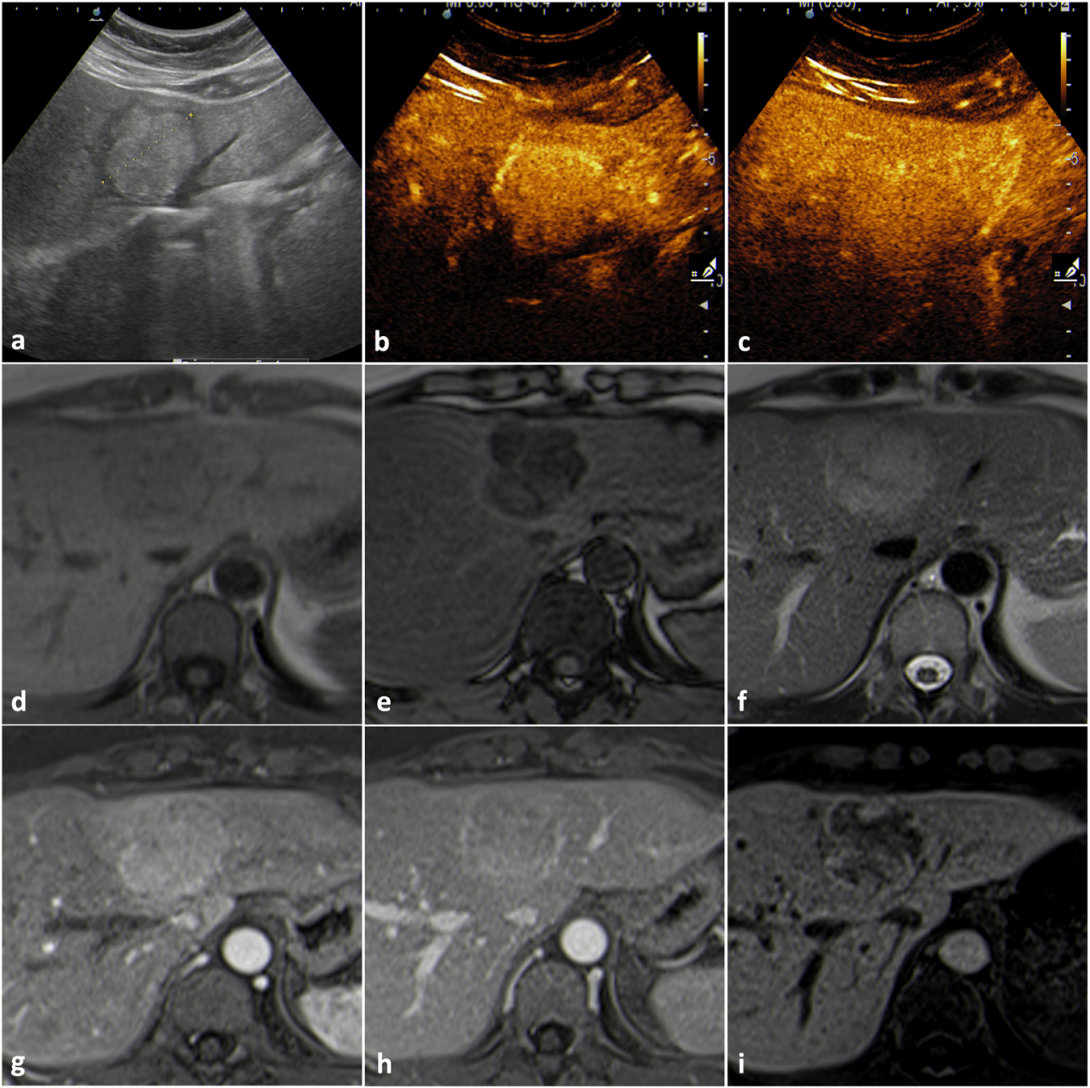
Inflammatory adenoma (biopsy-proven and resected). B-mode US (a) shows a lobulated hyperechoic mass surrounded by a hypoechoic halo. Contrast-enhanced US (b, c) shows homogeneous arterial enhancement without washout. On MRI, the lesion appears isointense on T1-weighted images (d) with a signal drop in the out-of-phase T1-weighted acquisition (e) and slightly hyperintense on T2-weighted images (f). The lesion shows homogeneous enhancement in the arterial phase (g), minimal washout in the portal venous phase (h) and appears hypointense in the hepatobiliary phase (i)
